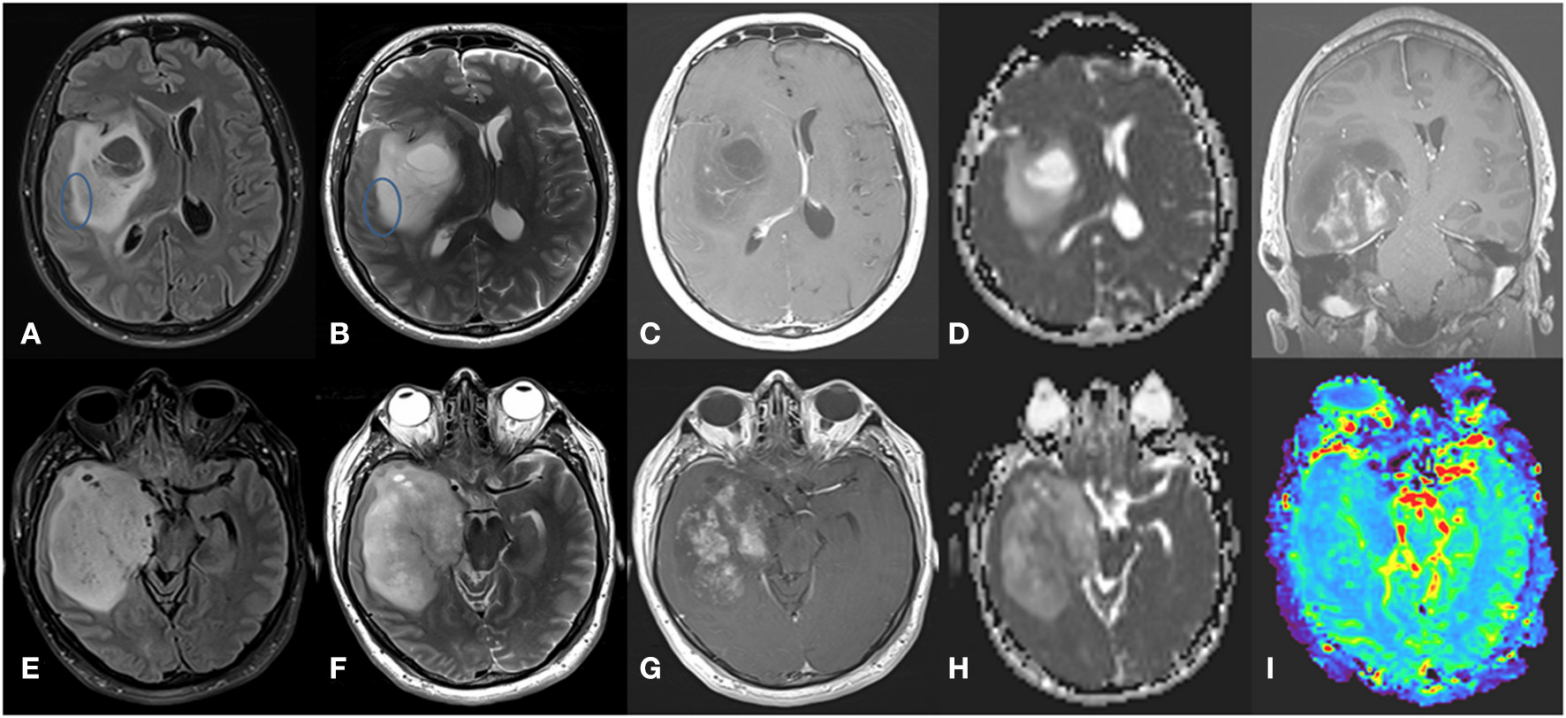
Grade 4 IDH-mutant astrocytoma. (A) axial FLAIR, (B) axial T2-weighted image, (C) axial T1-weighted image with contrast, and (D) ADC map in the superior aspect of the lesion, showing a large infiltrative and expansive insular lesion. Note the partial T2-FLAIR mismatch sign at its lateral margin (circle) and extensive NCE component with no or only subtle enhancement with high ADC values. These findings indicate IDH-mutant astrocytoma. In contrast, the inferior aspect of the lesion (E–H) has a more heterogeneous T2 signal with hypointense areas corresponding to low ADC values and elevated rCBV in perfusion map (I) and intense poorly delimited enhancement with small necrotic areas. These findings indicate a high-grade tumor.
